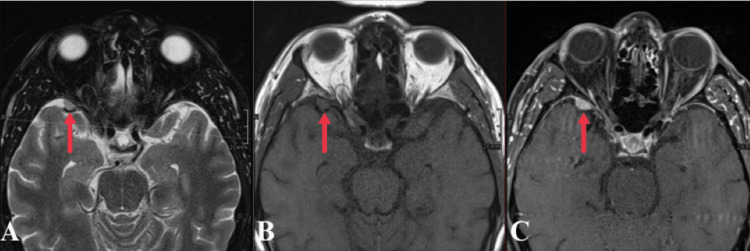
Axial T2 (A), T1 pre-contrast (B), and T1 post-contrast (C) MRI images demonstrate a T1/T2 isointense dural-based mass along the anterior middle cranial fossa measuring 10 x 8 x 10 mm with avid enhancement and dural tail
